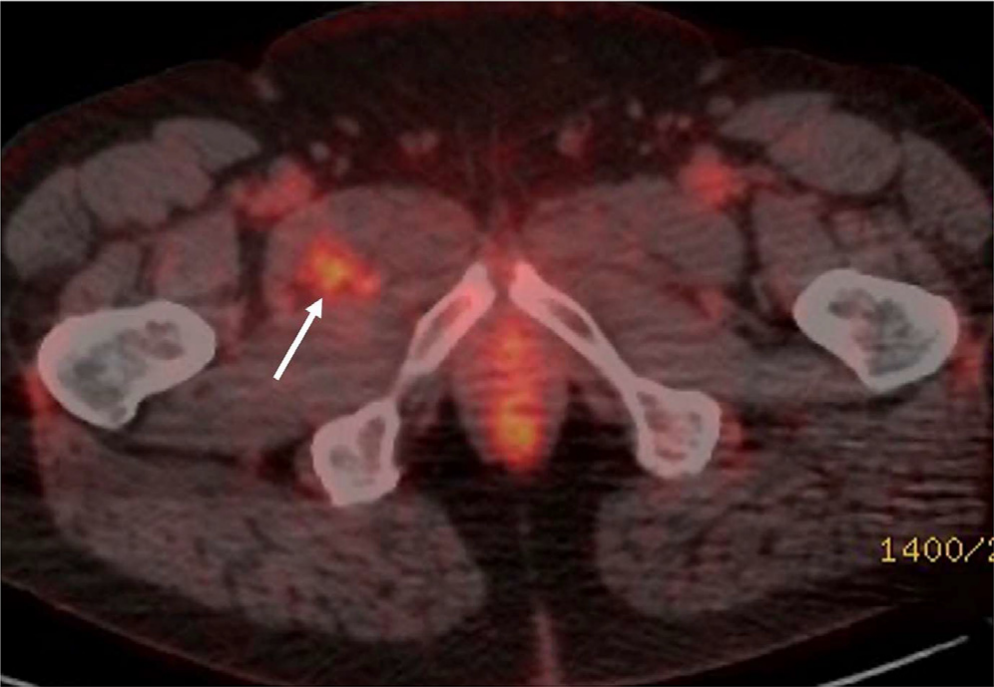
Selected PET-CT axial slice of the same patient demonstrates high FDG uptake in the right thigh anterior musculature (white arrow).
